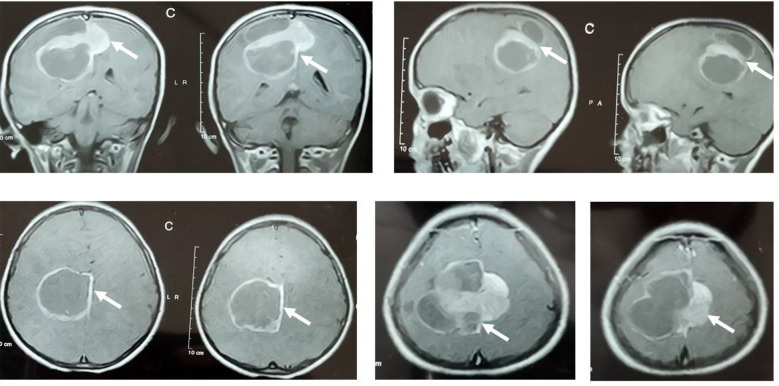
Case 3: MRI brain T1W post-contrast images: multi-lobulated heterogeneously enhancing, 5.5*6.7*6.3cm lobulated solid/cystic mass lesion in the right parafalcine region along the parietal lobe crossing midline and significantly compressing ipsilateral lateral ventricle and distal half of body of corpus callosum.
